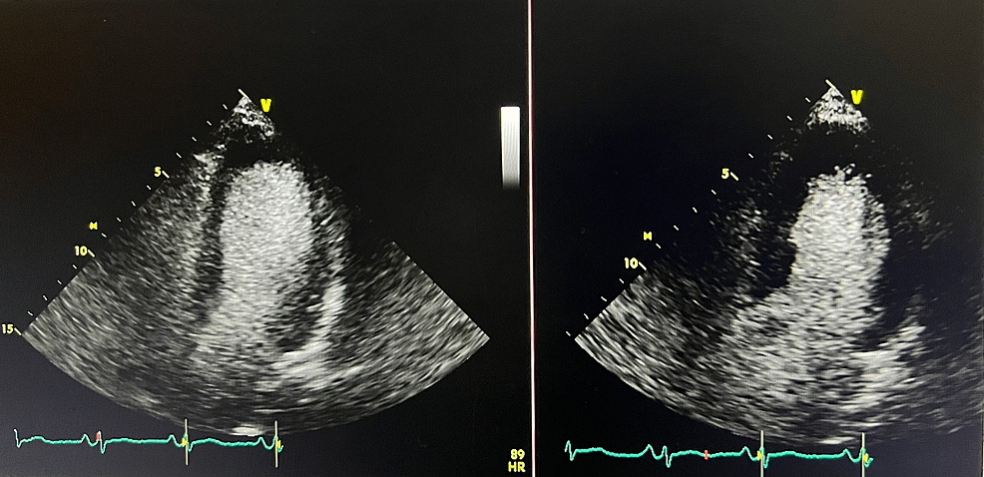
Transthoracic echocardiography four-chamber view showing normal EF at the end of systole and diastole, respectively EF; ejection fraction
